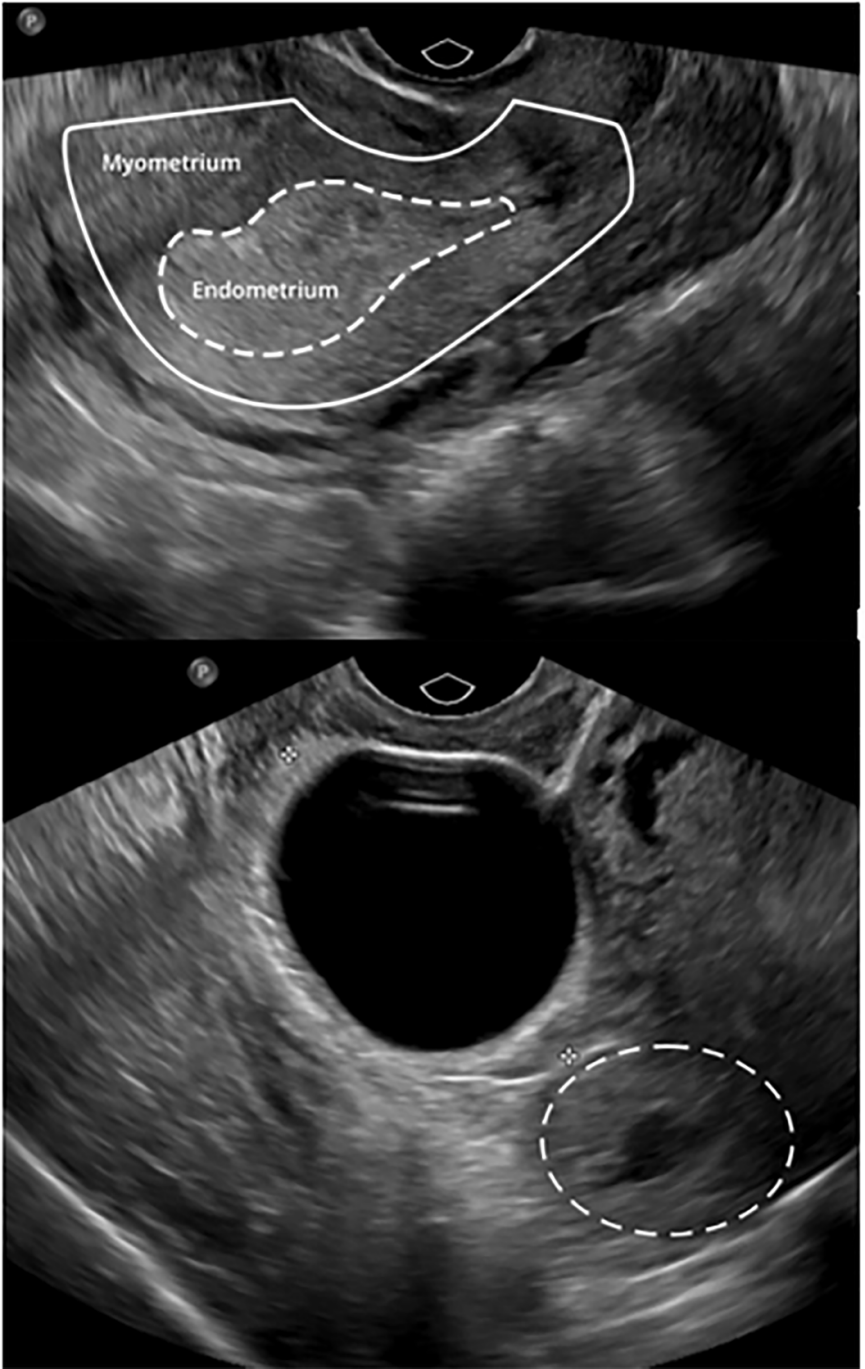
Initial transvaginal ultrasound six weeks prior to presentation, revealing an
